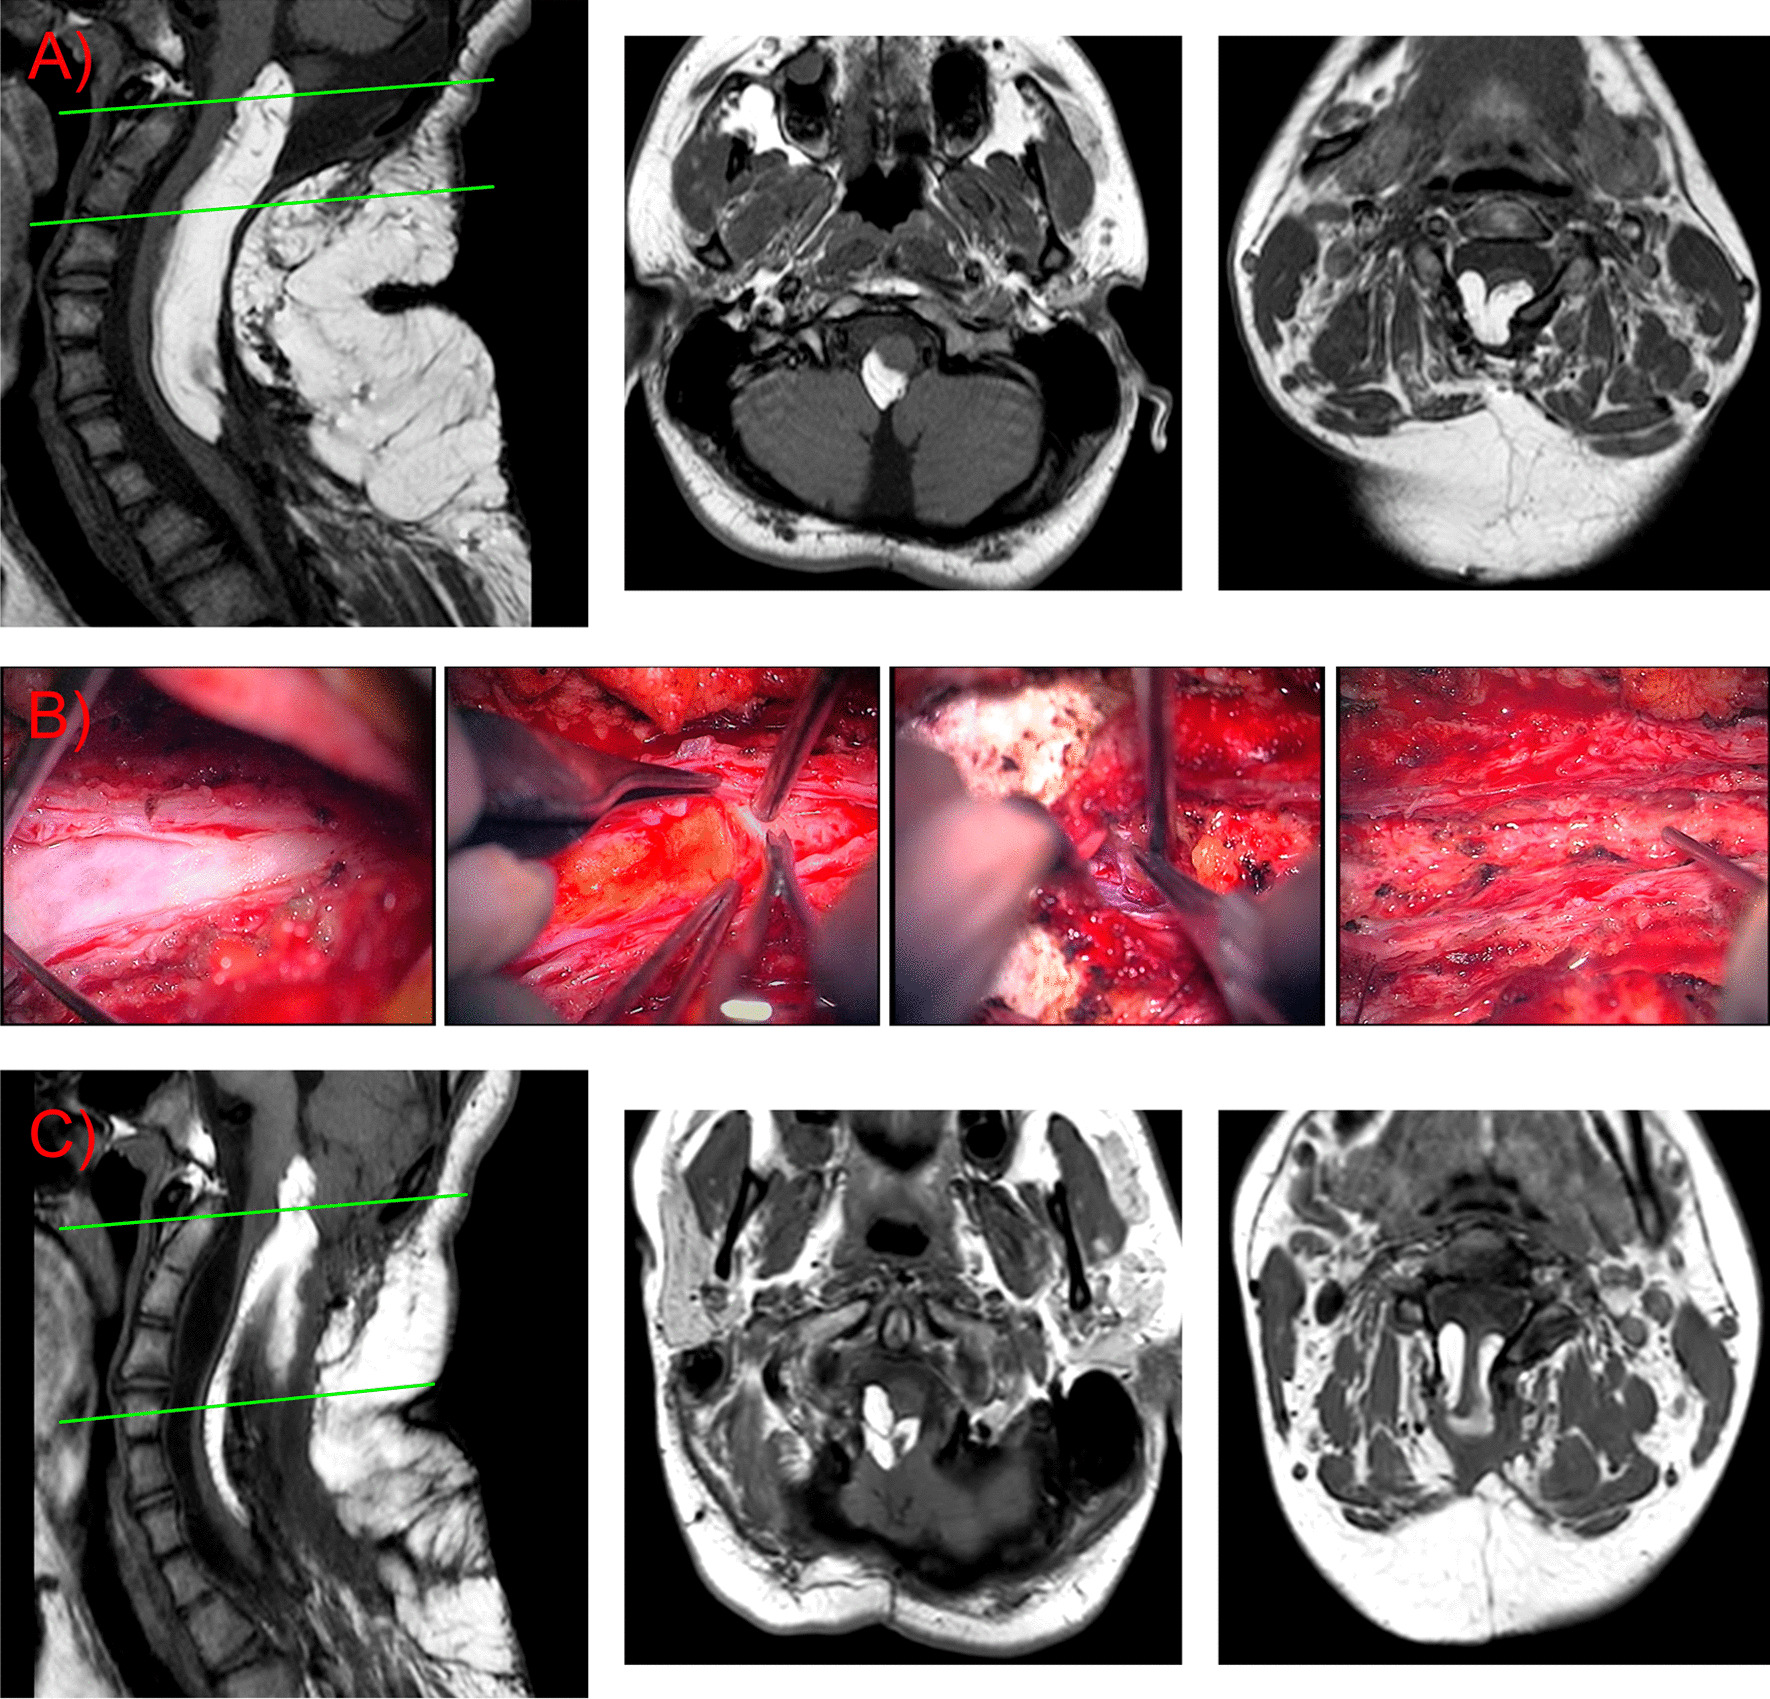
A Sagittal and axial Magnetic resonance images showing the cervicomedullary lipoma. Note the absence of laminas in the cervical vertebrae, a result of the previous surgical resections. B Intraoperative images. From left to right, we can see the arachnoid coat, the subpial lipoma, the cervicomedullary junction after lifting part of the lipoma, and finally, stimulation of residual tumor remains anchored to the posterior part of the medulla. C Postoperative MR images. The posterior lengthening of the medullary cord made resection of the tumor quite challenging. Green lines show the level where the sagittal magnetic resonance imaging is sliced to show the axial view
